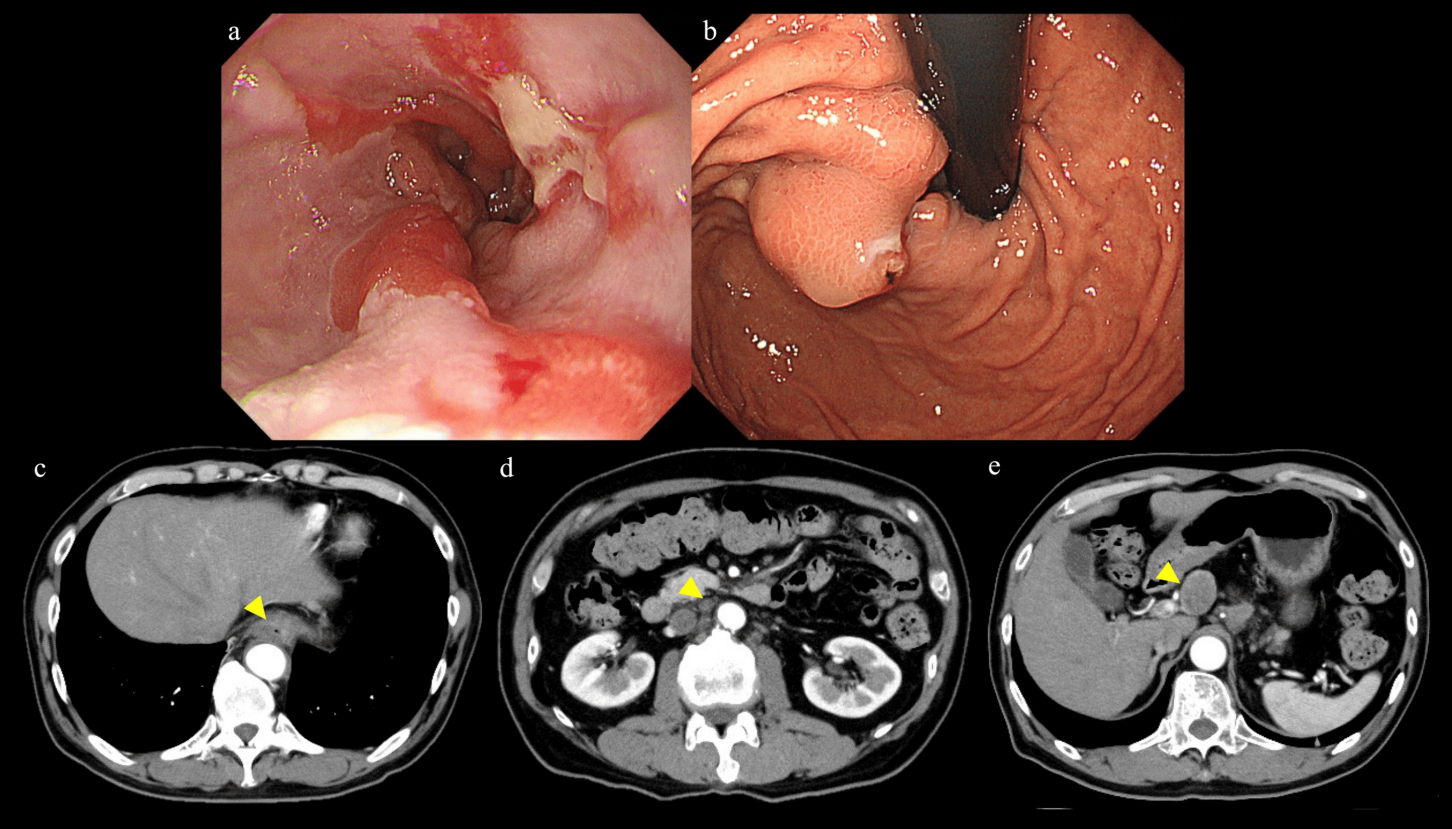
Post-treatment images (after eight courses of nivolumab therapy). (a, b) Upper gastrointestinal endoscopy showing mild shrinkage of the tumor. (c, d) CT showing that both the primary lesion and metastatic lymph nodes tended to shrink in size (yellow arrowhead). (e) Some lymph nodes (in the region of the anterior superior portion of the common hepatic artery) showed a tendency to increase in size (yellow arrowhead).
